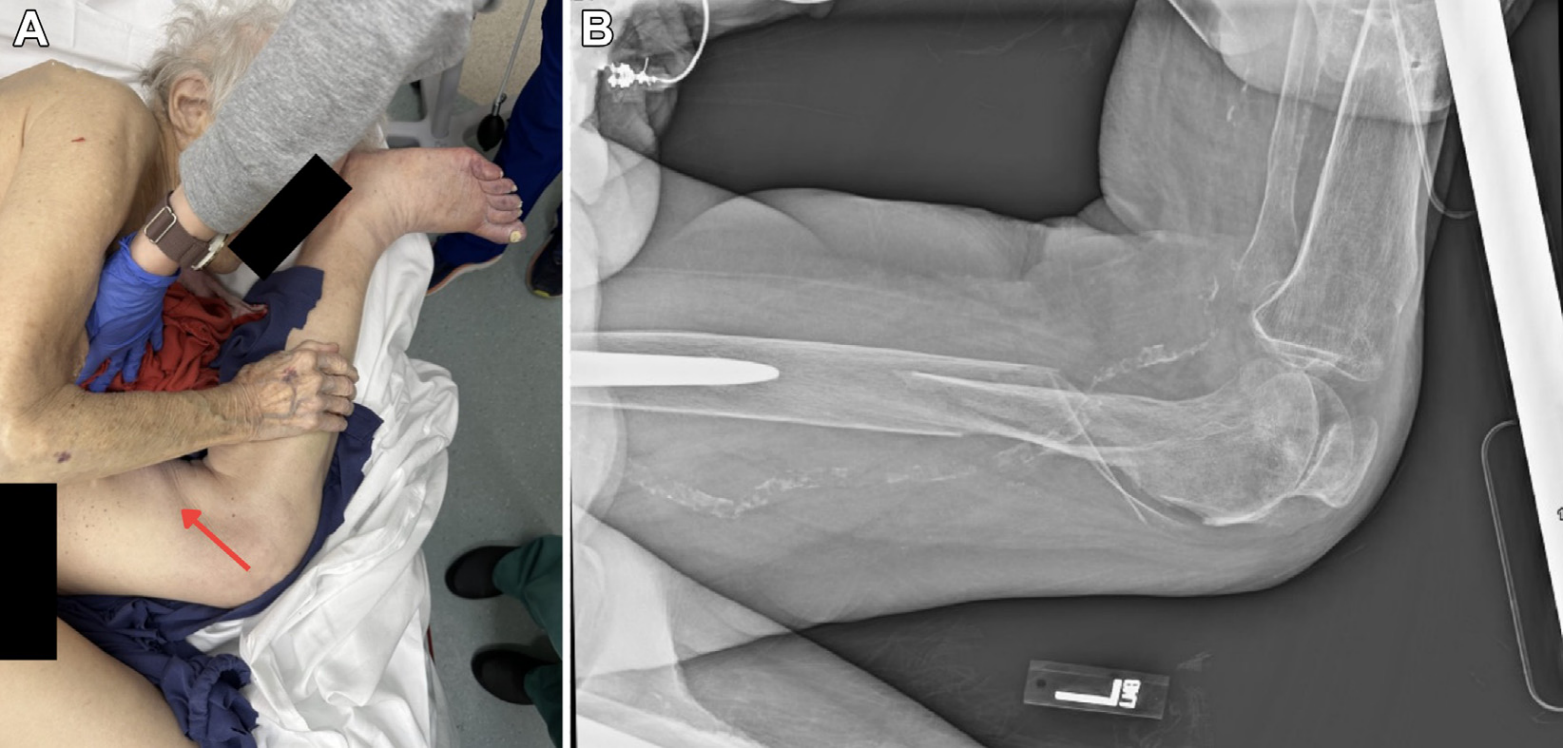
(A) A fall from the bed left the patient with rolled left lateral decubitus, and the left hip flexed with rotation at a mid-thigh deformity (red arrow) so that the lower leg was cephalad. A single-view lateral radiograph of the left femur (B) showed a laterally displaced, comminuted femoral shaft fracture.
